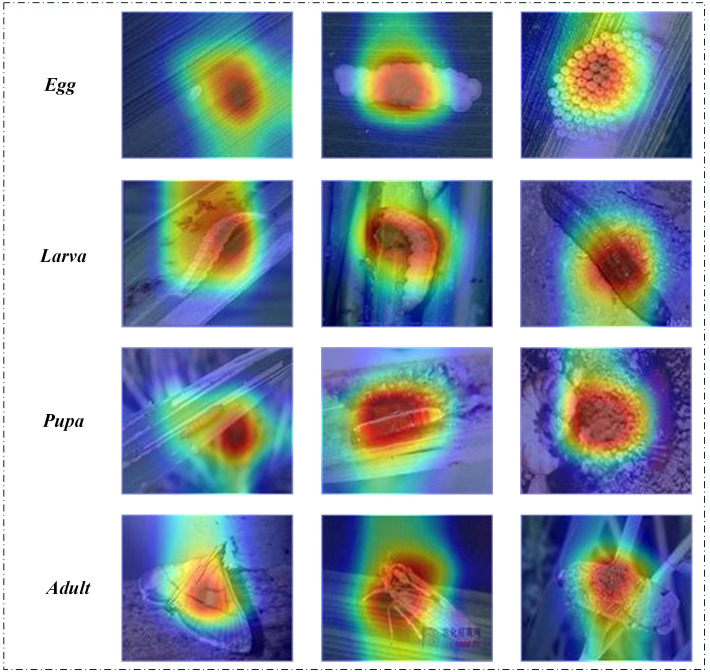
Grad-CAM visualization results of CT-PestNet for some samples from the IP102 dataset.
